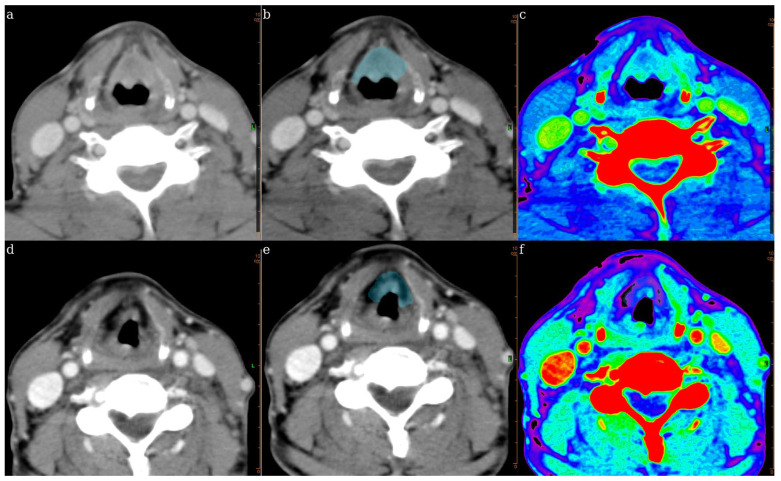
Representative axial CT images of a T3 laryngeal cancer patient before and after radiotherapy. Top row (a–c): Pre-treatment images showing (a) original CT, (b) tumor segmentation, and (c) texture-mapped color scale. Bottom row (d–f): Post-treatment images showing (d) original CT, (e) updated segmentation, and (f) post-treatment texture-mapped color scale. L, left side.
